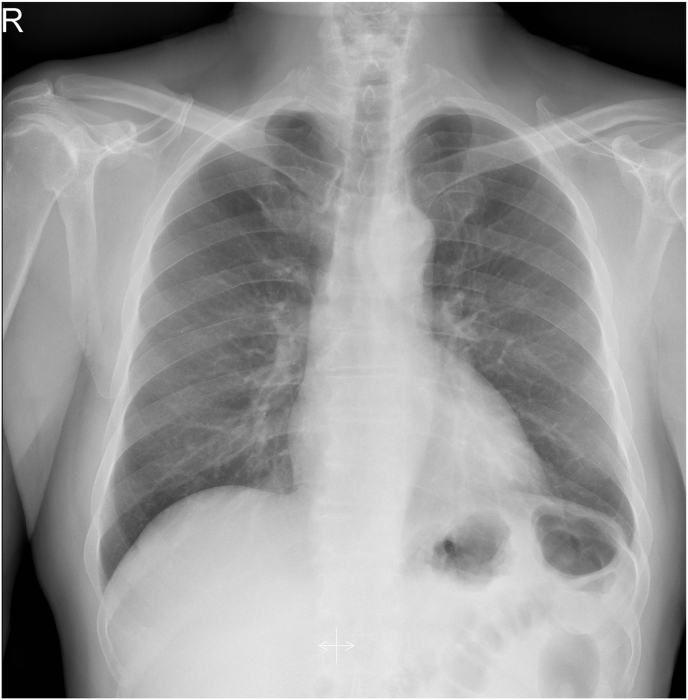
Chest radiograph showing a left lower thoracic opacity that obscure the left heart border.
